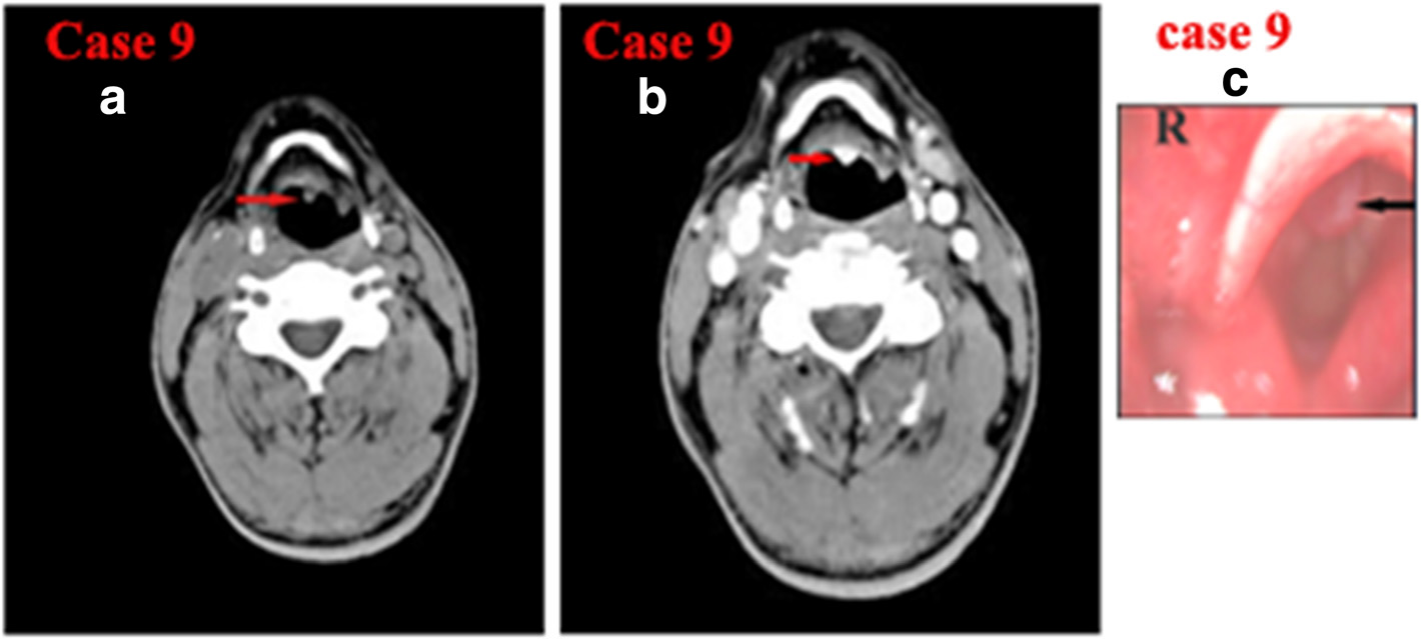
CT of laryngeal small-cell neuroendocrine carcinoma of Case 9. a) Noncontrast axial CT showing a small well-defined soft-tissue mass in the left epiglottic base not involving the bilateral vocal cord and laryngeal cartilages. b) Contrast-enhanced CT showing that the lesion was strongly enhanced. c) Laryngostroscopy showing a red smooth mass in the laryngeal surface of epiglottis.
